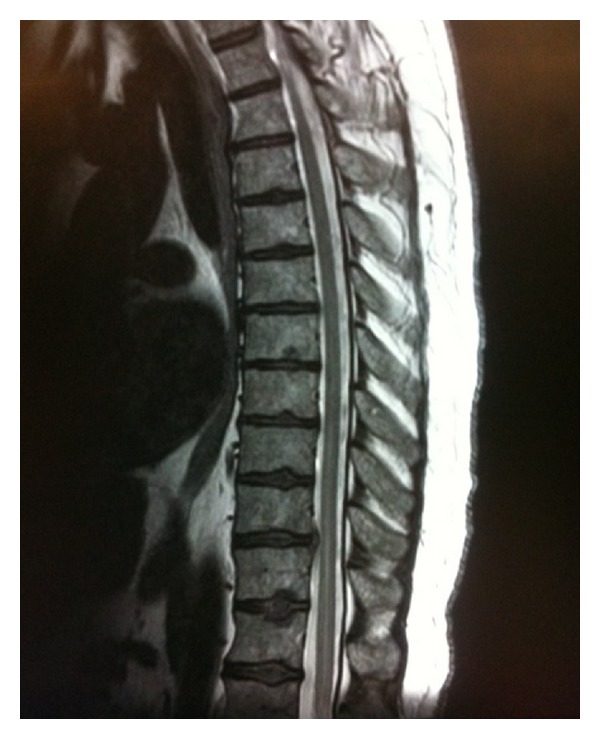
MRI thoracic spine showing acute spinal cord infarction. Sagittal T2-weighted MRI of the patient's thoracic spine demonstrating nonspecific central intrinsic signal hyperintensity of the spinal cord located in conus region with extension to T8 level. Multilevel thoracic disc disease at T1 and T10-T11 is also present.
